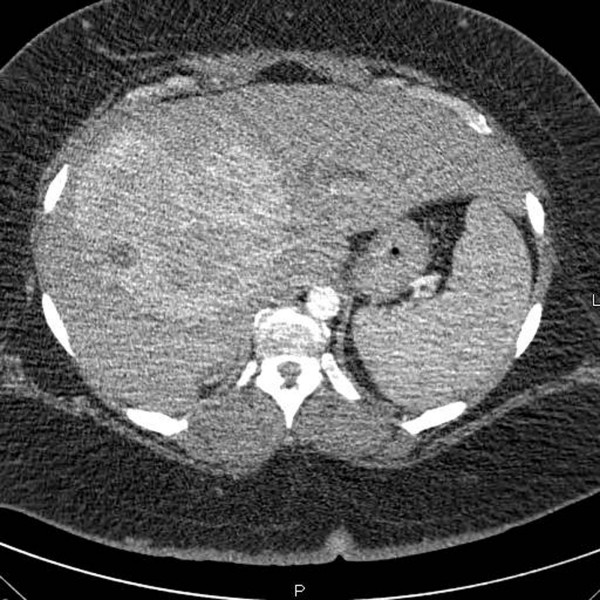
A triple-phase helical CT scan shows a 14-cm hypervascular mass involving the right lobe and the medial segment of the left lobe of the liver.
